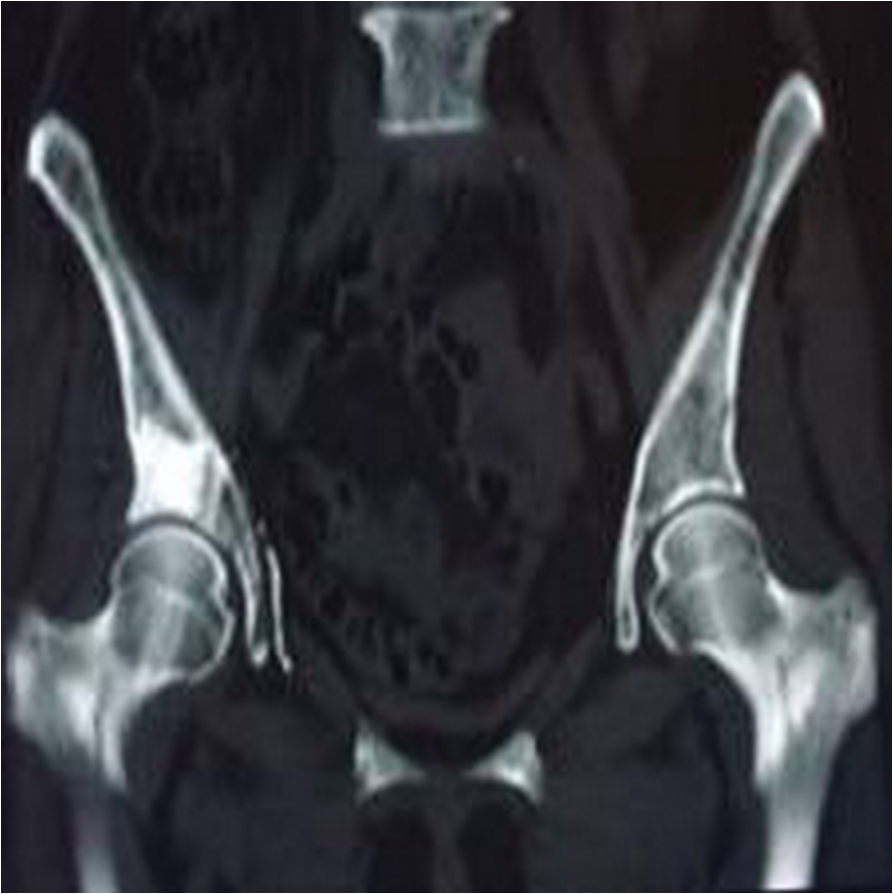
Postoperative CT shows bone cement filling of the cortical bone destruction area.
